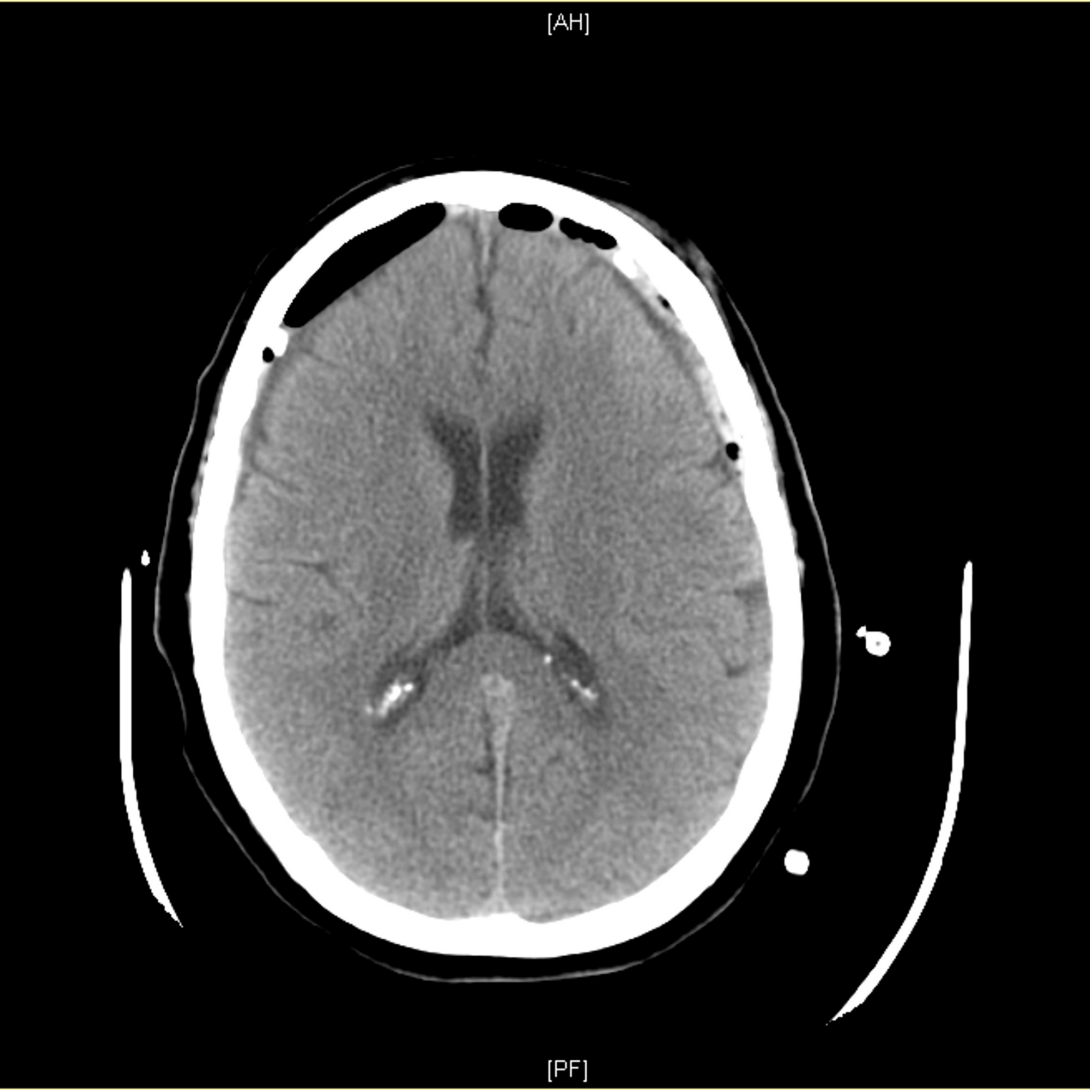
Postoperative head computed tomography (CT) scan reveals successful decompression of the brain bilaterally
